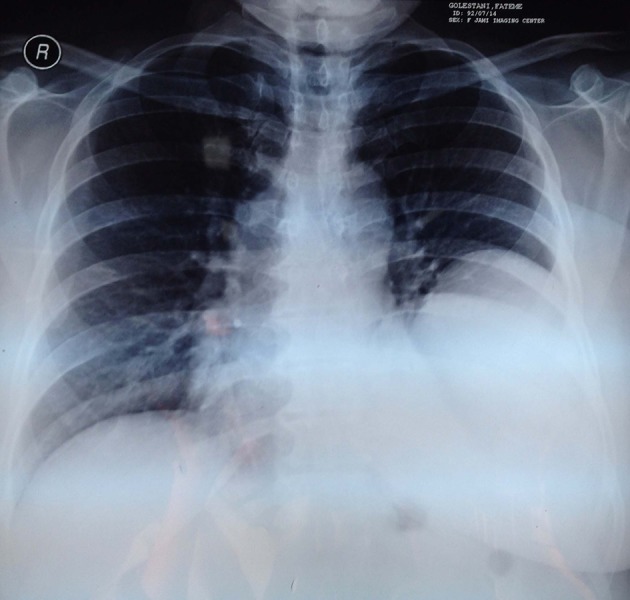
Chest Radiography on Admission
